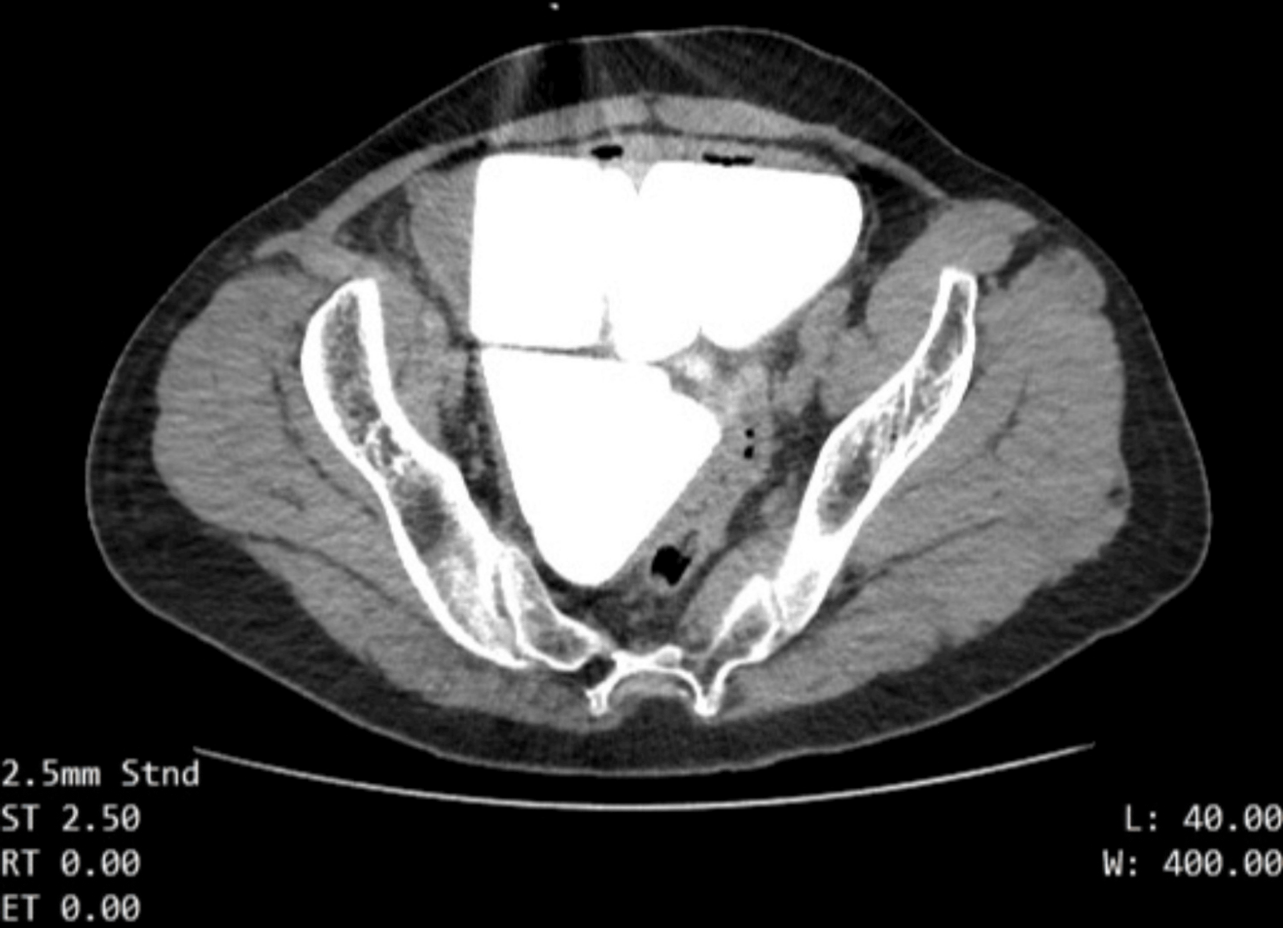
An axial CT image of bladder stones
